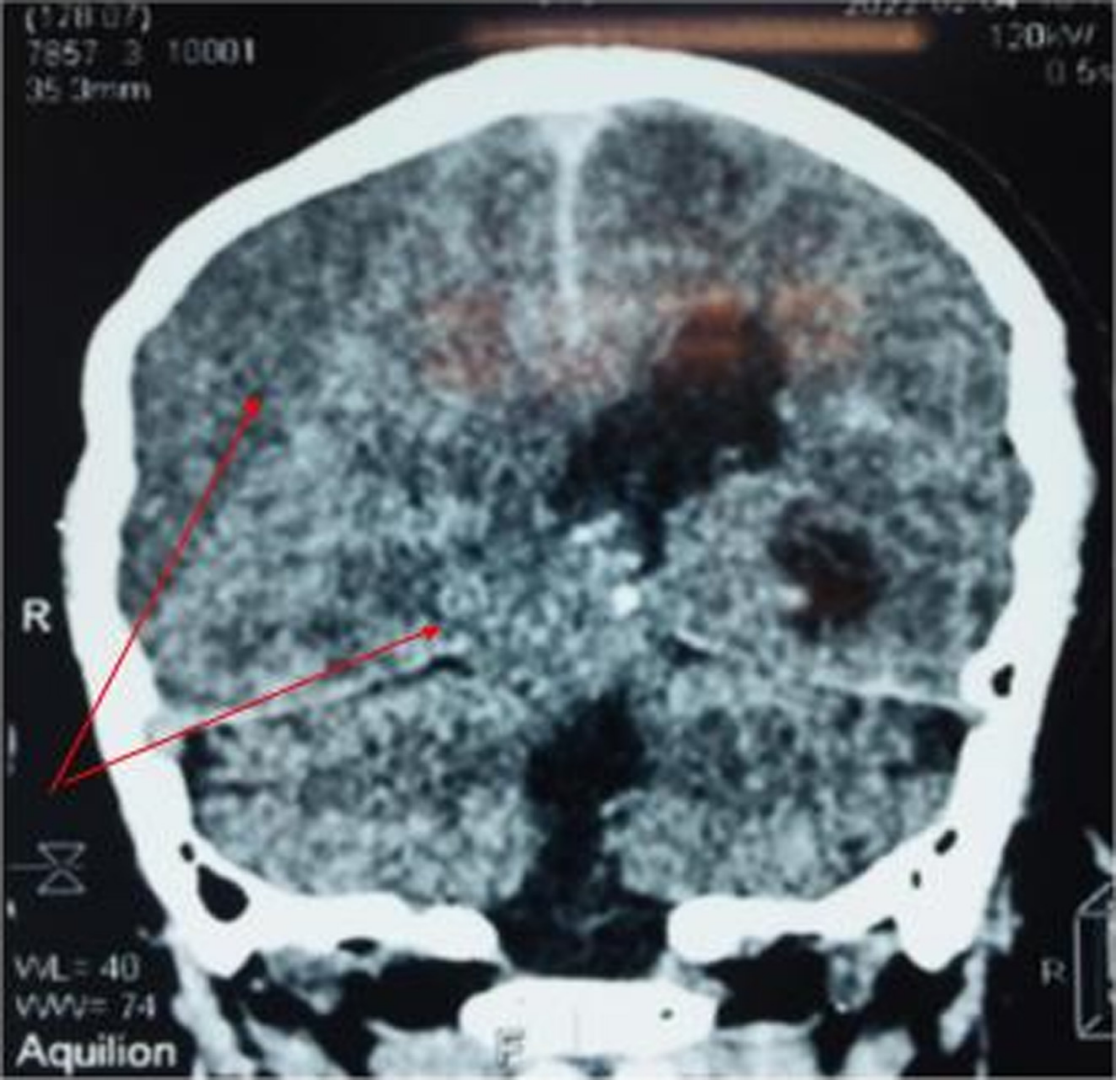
Cerebral CT image, coronal reconstruction showing a large right hemispheric chronic subdural hematoma with a temporal hernia.
